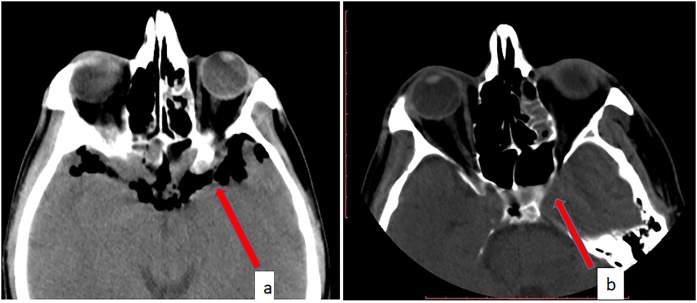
a. Noncontrast axial cranial CT showing pneumocephalus in the frontal region of the brain and in the subarachnoid space (red arrow). b. Noncontrast axial cranial CT showing disappearance of pneumocephalus at 72 h (red arrow).
